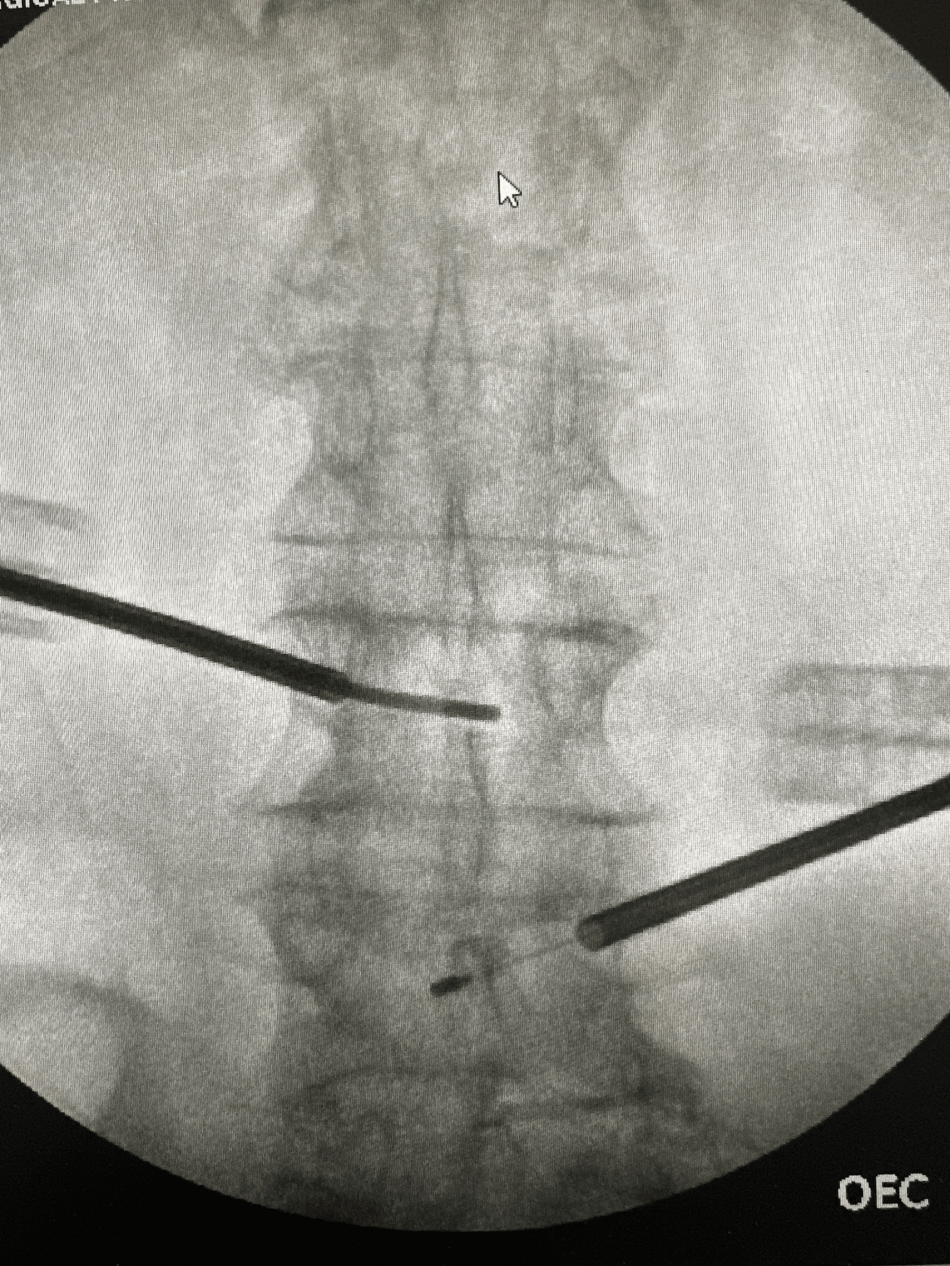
Fluoroscopic image showing transpedicular probe placement for basivertebral nerve ablation at the L3-S1 vertebral endplate with radiographic evidence of Modic endplate changes.
